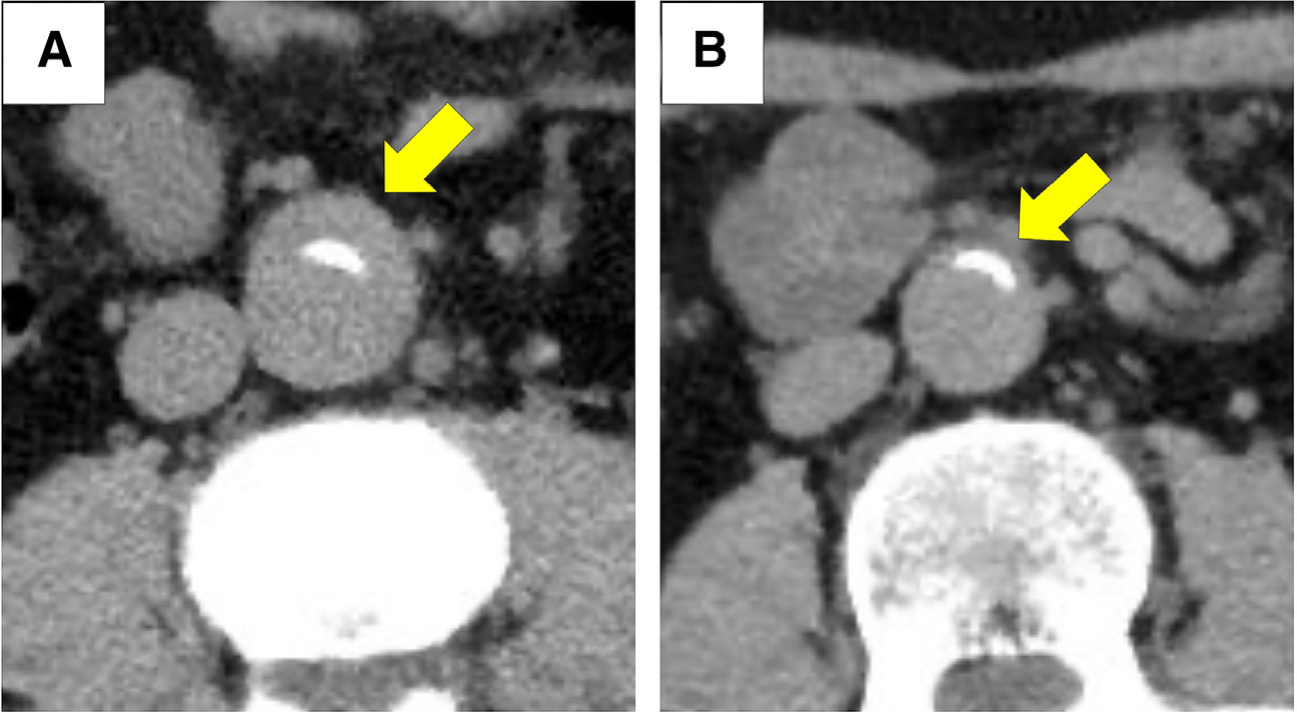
Changes in Abdominal Aorta Diameter in Computed Tomography (A) Two years after the initial visit, the diameter enlarged to 34 mm (arrow). (B) On the second month of corticosterone therapy, the wall thickening disappeared (arrow).
